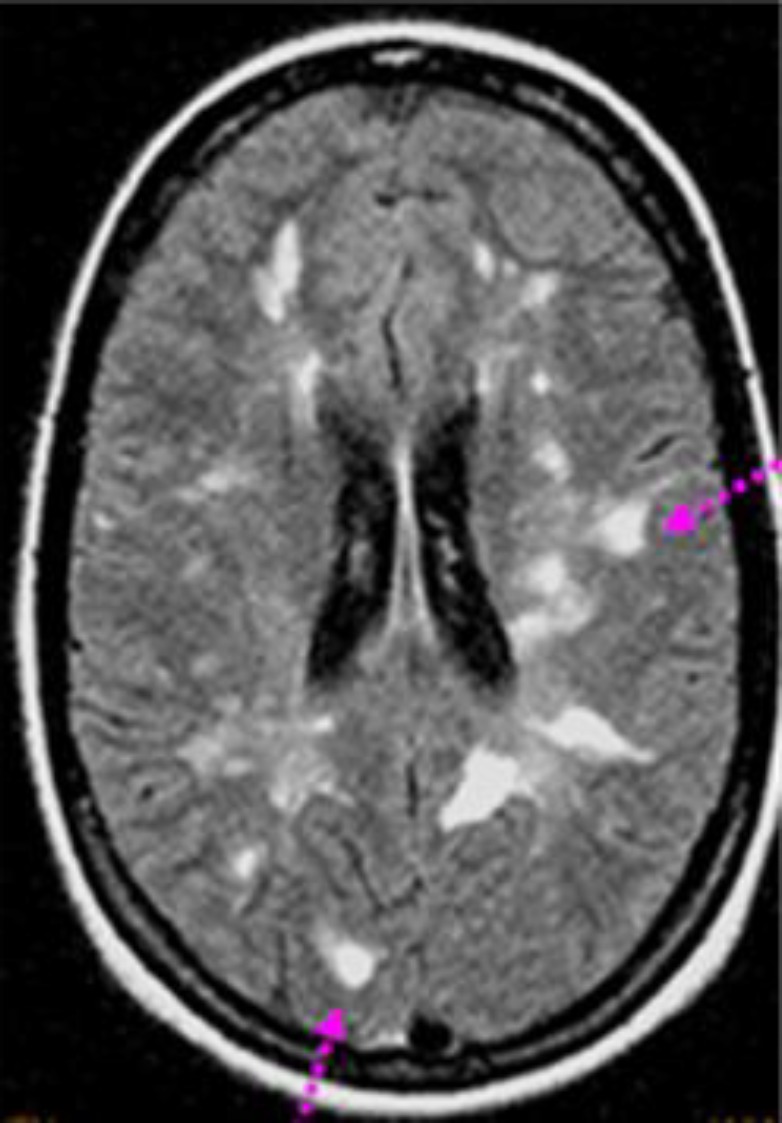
Cerebral MRI showed multiple enhancing lesions of bulb, cerebellar peduncles and extensive to the cervical spinal cord
